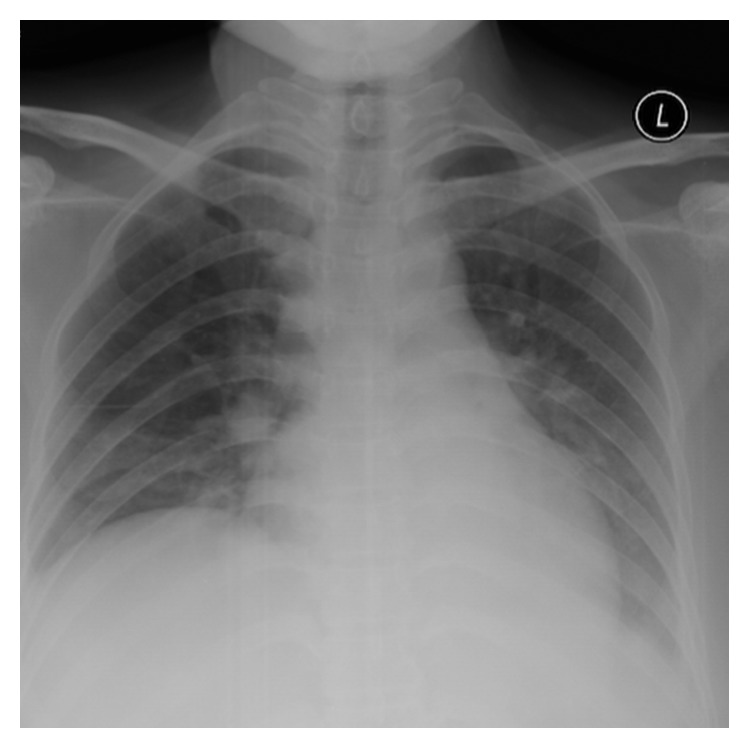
Chest radiograph revealed bilateral perihilar opacities which could represent enlarged hilar nodes. There was no evidence of fibrosis, pleural effusion, or infective changes.
